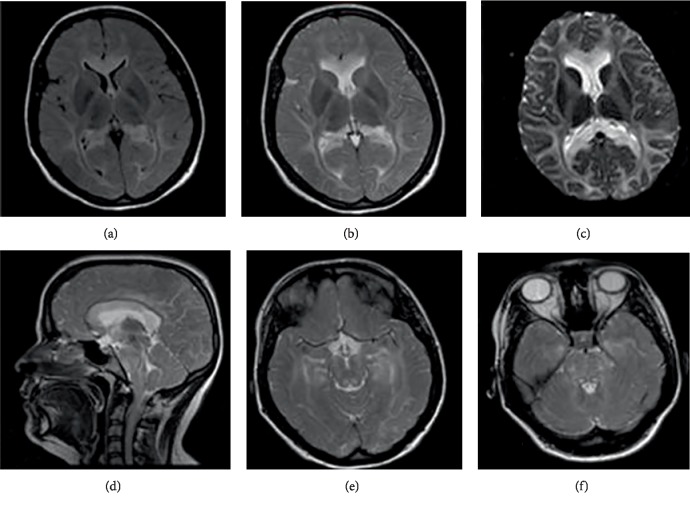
(a) Axial FLAIR showed hyperintense signal in supratentorial white matter. (b) Axial T2 showed hyperintense signal in supratentorial white matter. (c) Axial Diffusion showed restricted diffusion at the entire corpus callosum and at the white matter. (d) Sagittal T2 showed hyperintense signal in supratentorial white matter and also in the brainstem. (e) Axial T2 showed hyperintense signal at the mesencephalon. (f) Axial T2 showed hyperintense signal at the pons.
